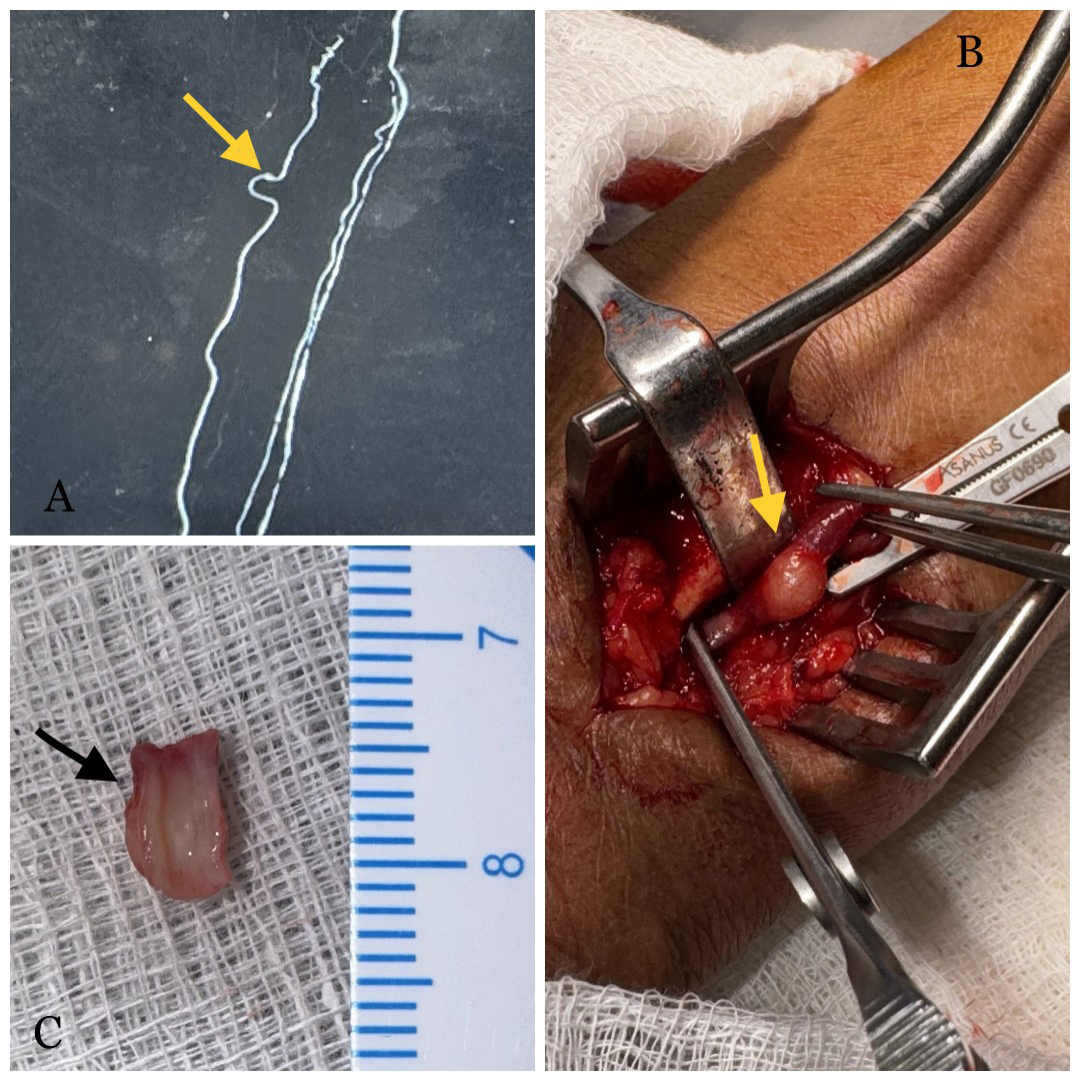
(A) CT angiogram with volume rendering of the right forearm region showing a kink in the ulnar artery with aneurysmal dilatation of the ulnar artery (arrow); (B) an intraoperative image showing aneurysmal dilatation of the ulnar artery with proximal and distal segments clamped, followed by excision and repair (arrow); (C) the excised aneurysmal segment of the ulnar artery, with the cut section revealing a healthy intima within the excised segment (arrow).
